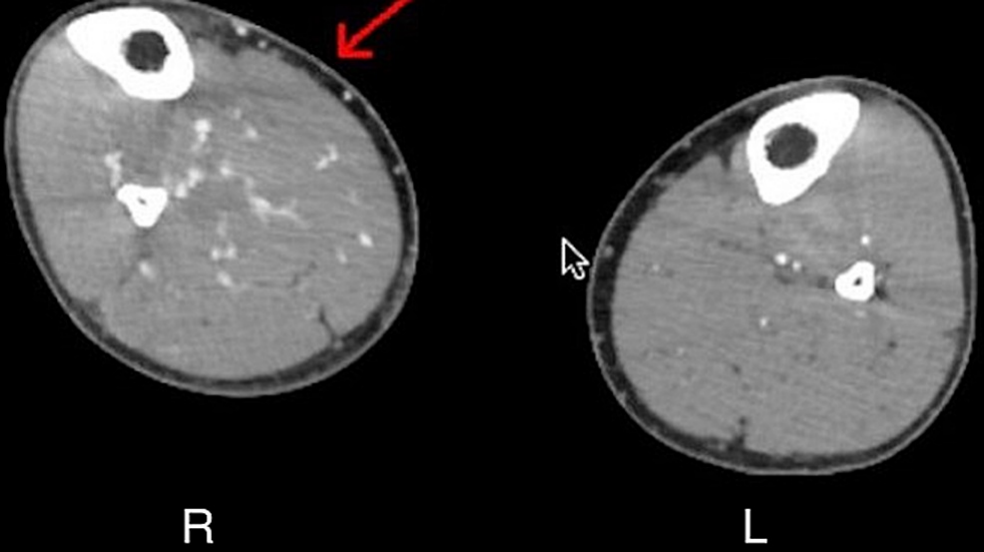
CT angiography with contrast of the lower extremities showing multiple contrast-enhanced receiving veins in the right extremity compared to the left extremity at the same level
